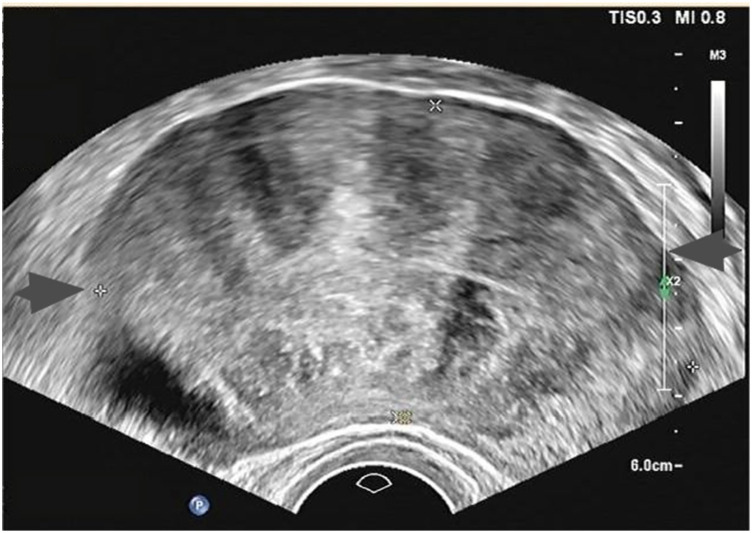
Ultrasound showed pelvic mass.
